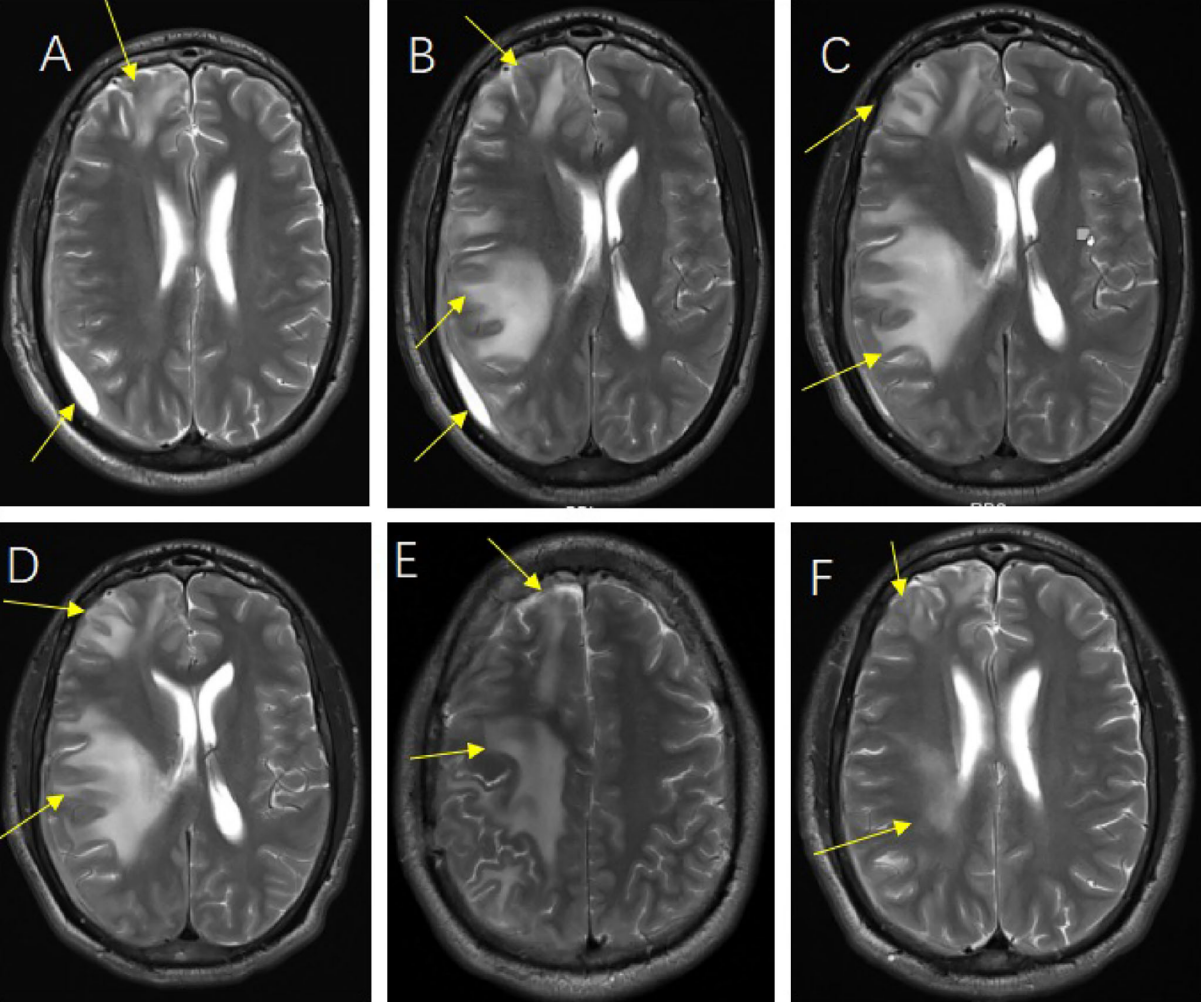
The follow-up head magnetic resonance imaging (MRI) image of post-operation. Head MRI on the 5th day after operation (A), Head MRI on the 14th day after operation (B), Head MRI on the 23rd day after operation (C), Head MRI on the 30th day after operation (D), Head MRI at the time of discharge (E, F).
